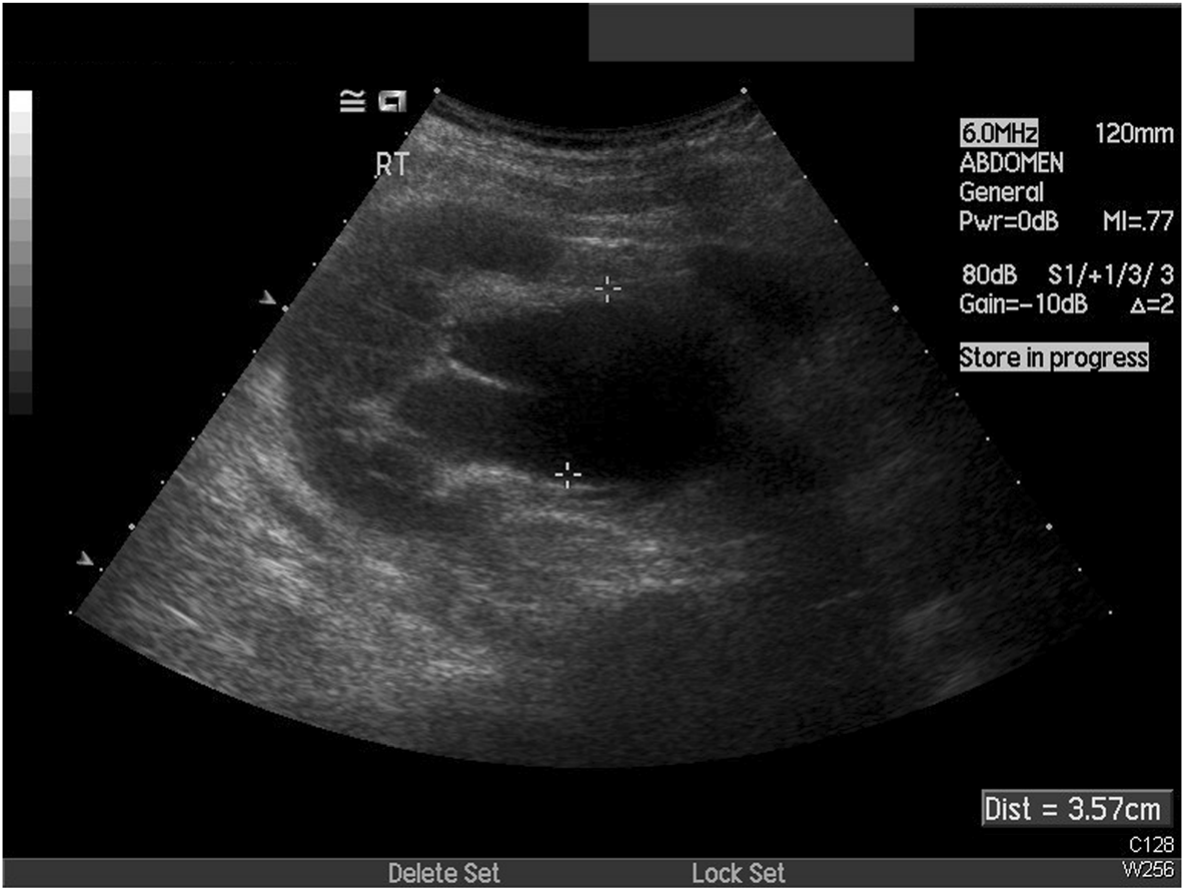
Ultrasound with hydronephrosis and dilated pelvis.
